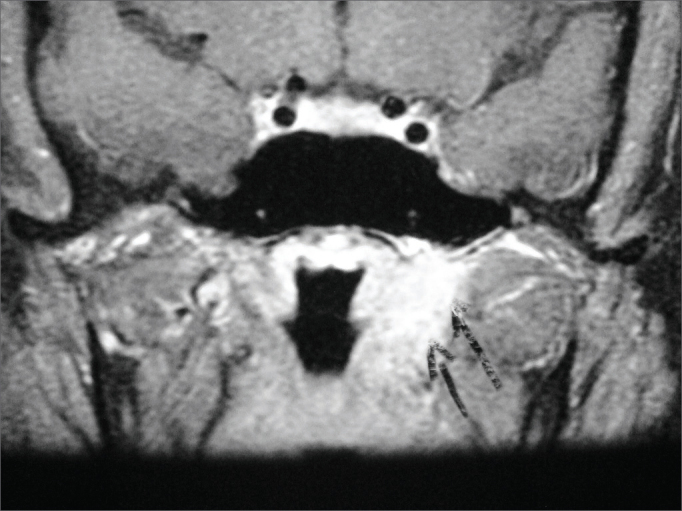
T1-weighed head MRI scan, coronal view after contrast injection; expansive injury seen on the left side wall f the rhinopharynx consistent with reactive lymphnode. The arrows indicate shifting of adjacent parapharyngeal fat.
